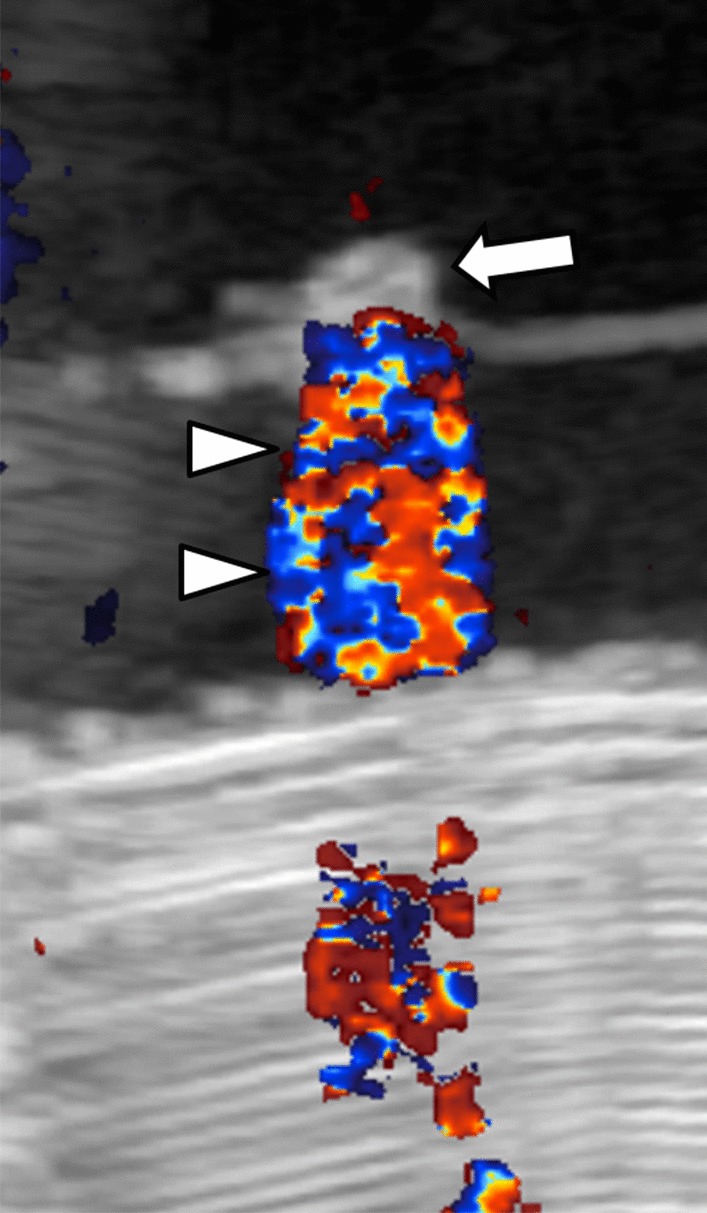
In vitro ultrasound of xanthine urinary stone: stone (arrow) shows posterior twinkle artifact (arrowheads) on color Doppler imaging
